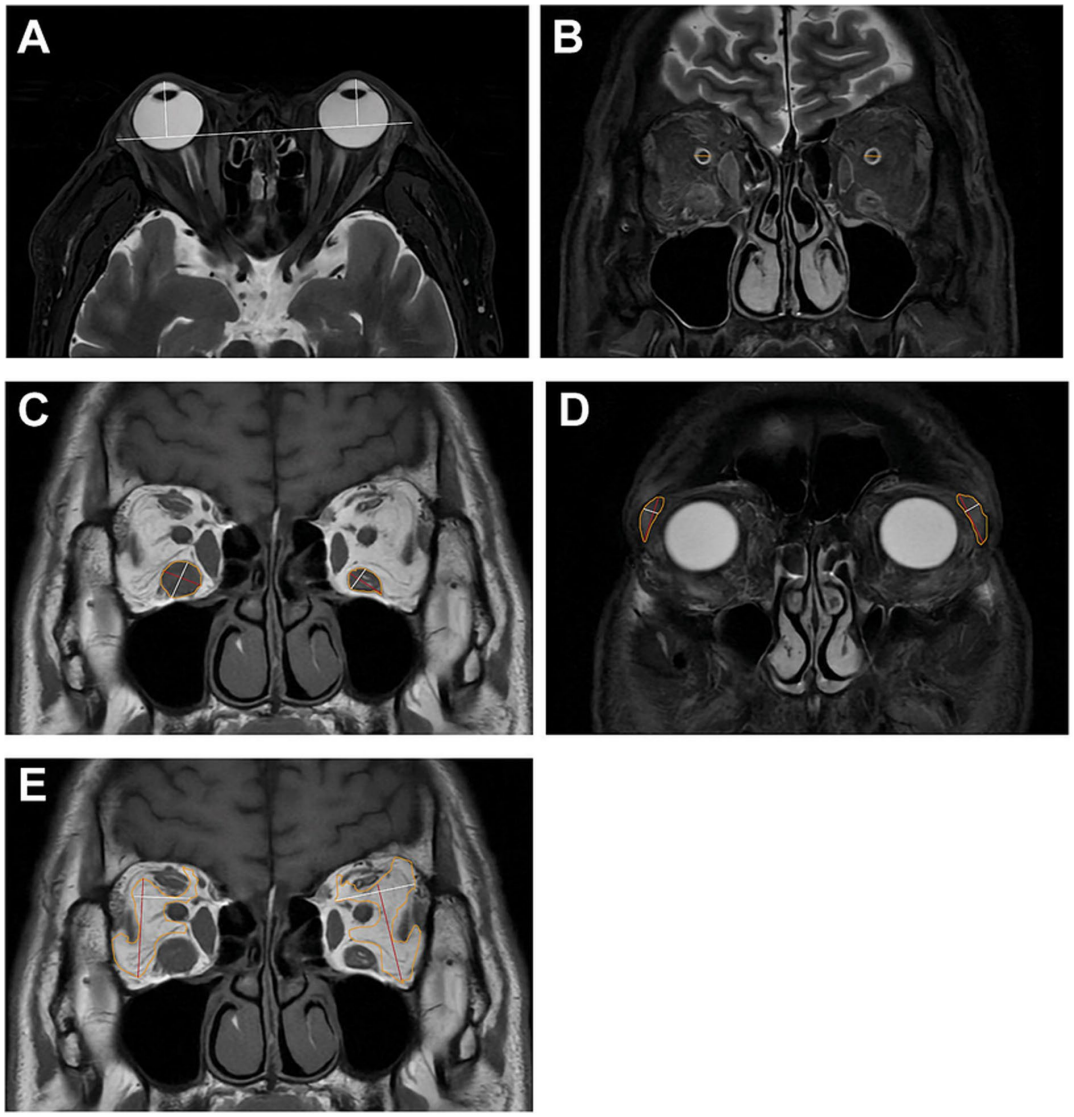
Measurement of MRI proptosis, optic nerve sheath diameter, maximum coronal length (MCL), and width (MCW) of the IR, LG, and OF. (A) MRI proptosis was defined as the perpendicular distance from the corneal eminence to a reference line connecting the most anterior points of the bilateral lateral orbital rims, measured on an axial T2 STIR image. (B) ONSD on a coronal T2 STIR image. (C–E) MCL (red line) and MCW (white line) of the inferior rectus (IR), lacrimal gland (LG), and orbital fat (OF).
